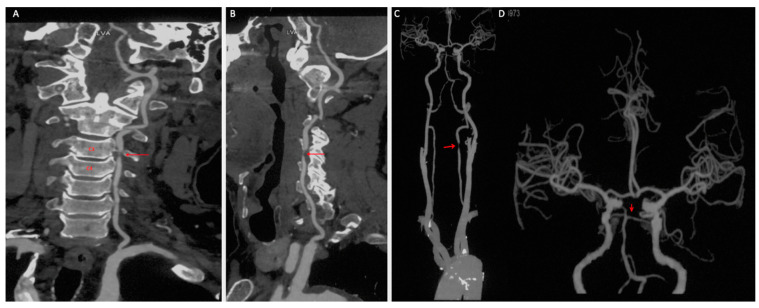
Target vertebral artery anatomy before endovascular intervention. (A,B) Computed tomography angiography of the left V2 segment before intervention. Dominant left vertebral artery V2 segment severe stenosis ((A–C), red arrow) and an undeveloped left posterior cerebral artery, together with incomplete circle of Willis, are shown ((D), red arrow).
